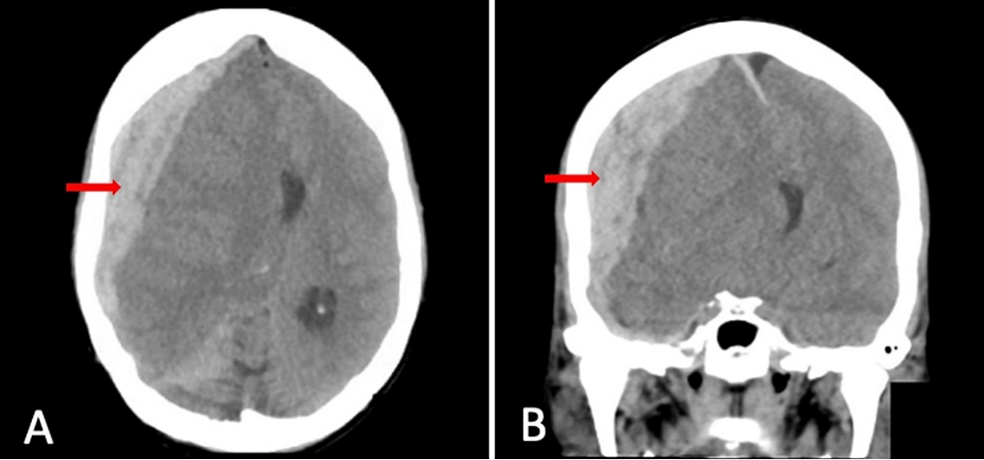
Non-contrast CT head revealing a 2 cm right-sided acute SDH (indicated by red arrows) with approximately 2.1 cm midline shift and subfalcine herniation SDH: Subdural hematoma, A: Axial view, B: Coronal view
